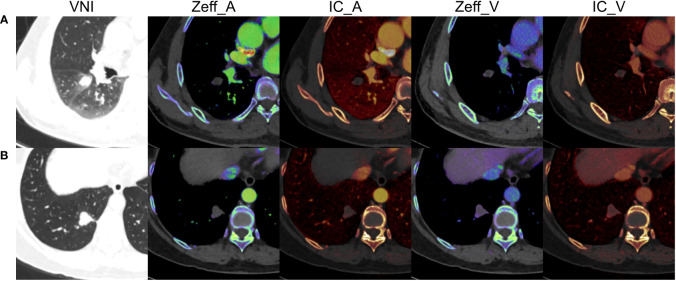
The representative dual-energy computed tomography images of solid pulmonary nodules. (A) A 49-year-old male with inflammation, diameter = 14.9 mm, volume = 1.949 cm^3^, Zeff_A = 7.57, IC_A = 0.10 mg/mL, NIC_A = 1.15%, Zeff_V = 7.80, IC_V = 0.50 mg/mL, NIC_V = 10.00%. (B) A 66-year-old female with adenocarcinomas, diameter = 18.8 mm, volume = 3.832 cm^3^, Zeff_A = 8.19, IC_A = 1.30 mg/mL, NIC_A = 10.92%, Zeff_V = 8.33, IC_V = 1.70 mg/mL, NIC_V = 27.42%. VNI, virtual non-enhanced images; Zeff_A, effective atomic number in arterial phase; IC_A, iodine concentration in arterial phase; NIC_A, normalized iodine concentration in arterial phase; Zeff_V, effective atomic number in venous phase; IC_V, iodine concentration in venous phase; NIC_V, normalized iodine concentration in venous phase.
